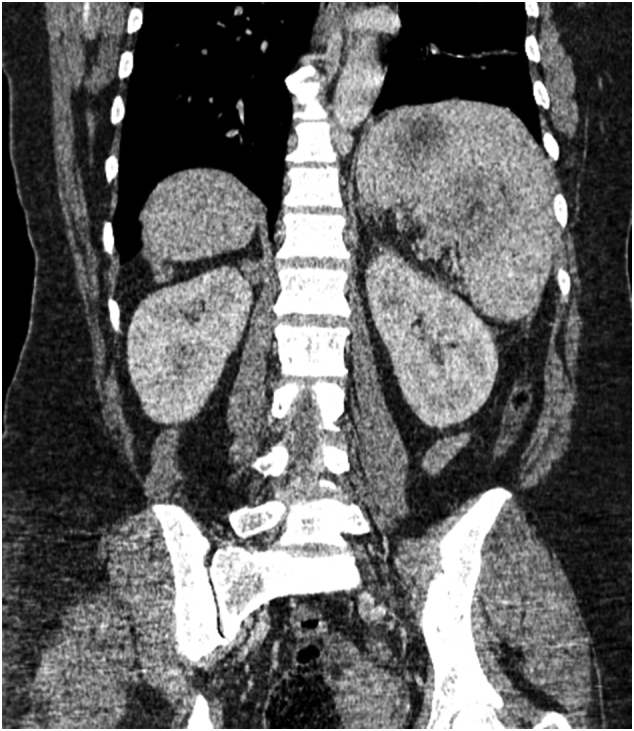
Coronal CT scan showing massive splenomegaly and lymph nodes in the splenic hilum.
